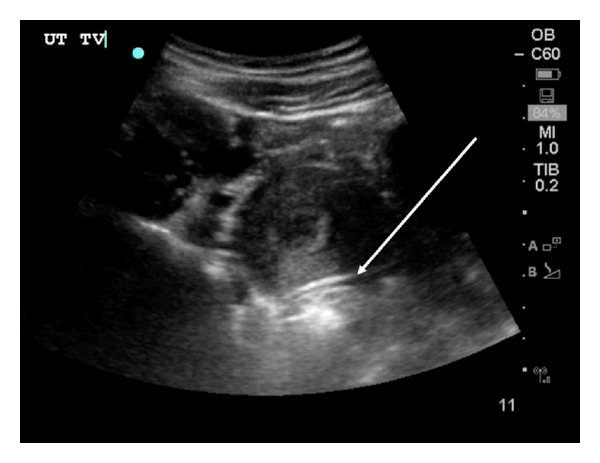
Utilizing a Sonosite C60 2–5 MHz curvilinear transducer, this transabdominal ultrasound image displays an empty uterus with a thin sliver of hypoechoic fluid posterior to the uterine wall (arrow).
